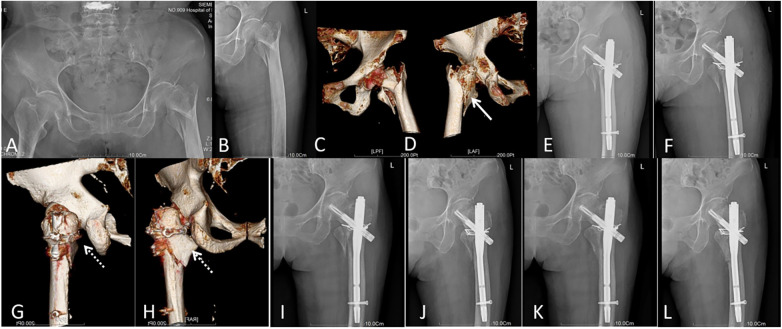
Augmented fixation case: A 73-year-old female sustained a left A2.3 intertrochanteric fracture with type 3 coronal fragments (A–D) following a ground-level fall. The patient underwent cable-plate augmentation combined with PFNA fixation (E–H). Weight-bearing ambulation with walker assistance commenced on postoperative day 2. Two-month postoperative radiographs revealed abundant callus formation at the fracture site (I,J), with complete fracture union confirmed at the 12-month follow-up (K,L). The solid arrows delineate the large displaced coronal fragment, whereas the dashed arrows indicate the cable-plate augmentation achieving anatomical reduction and stable fixation.
